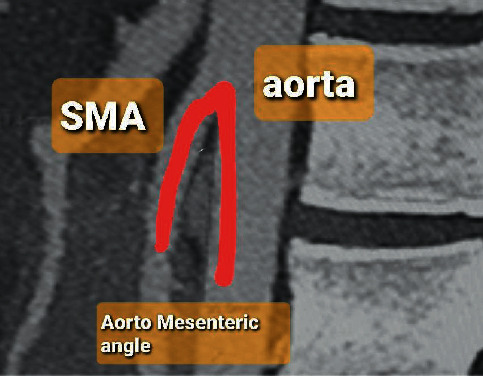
CT scan, sagittal view, showing an aortomesenteric angle of 13°.
